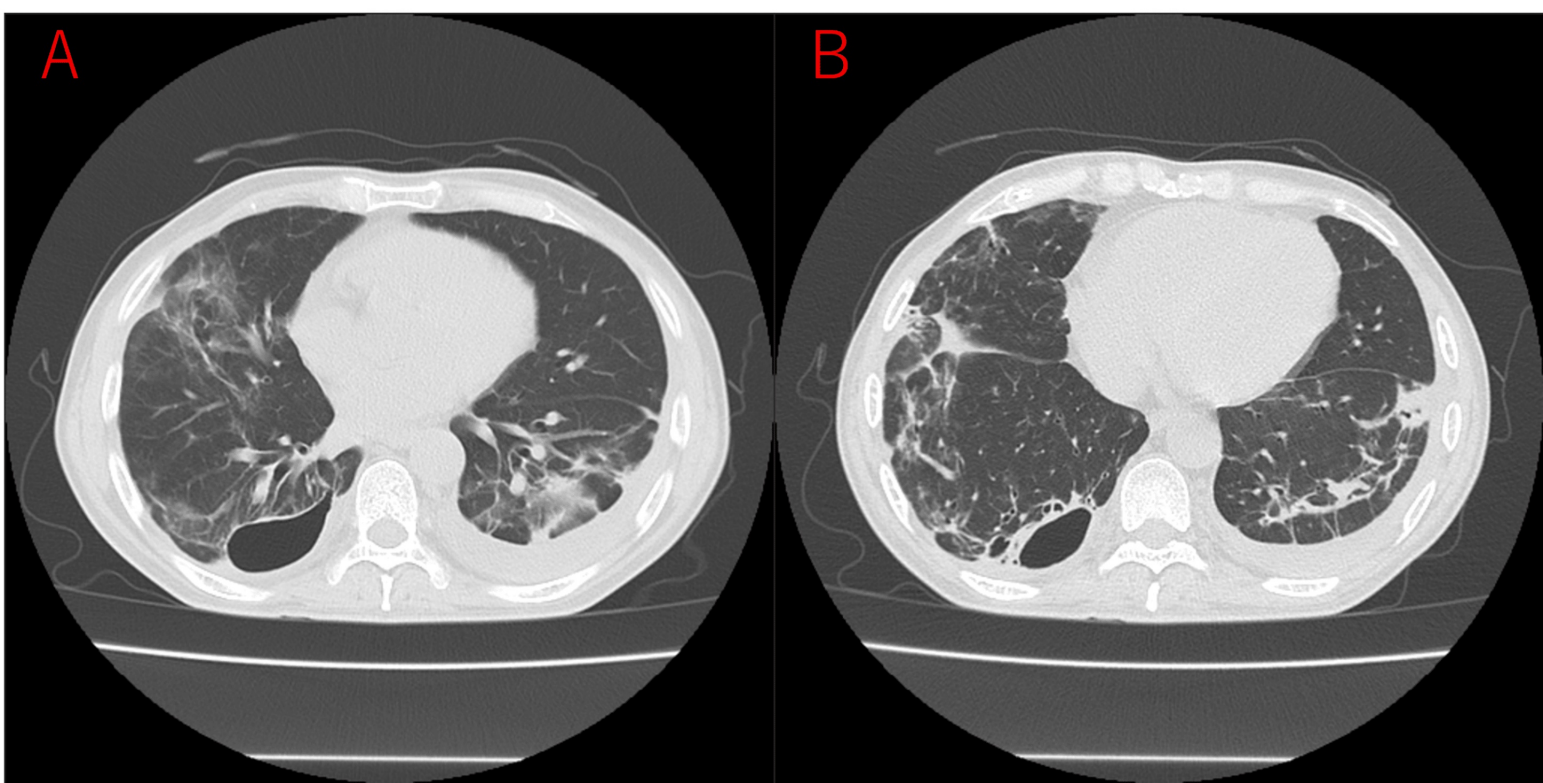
Follow-up chest computed tomography scan at discharge Axial (A, B) view shows considerable resolution of the previously observed pulmonary infiltrates and cavitary lesions in the right lung compared to the admission computed tomography (Figure 3).
